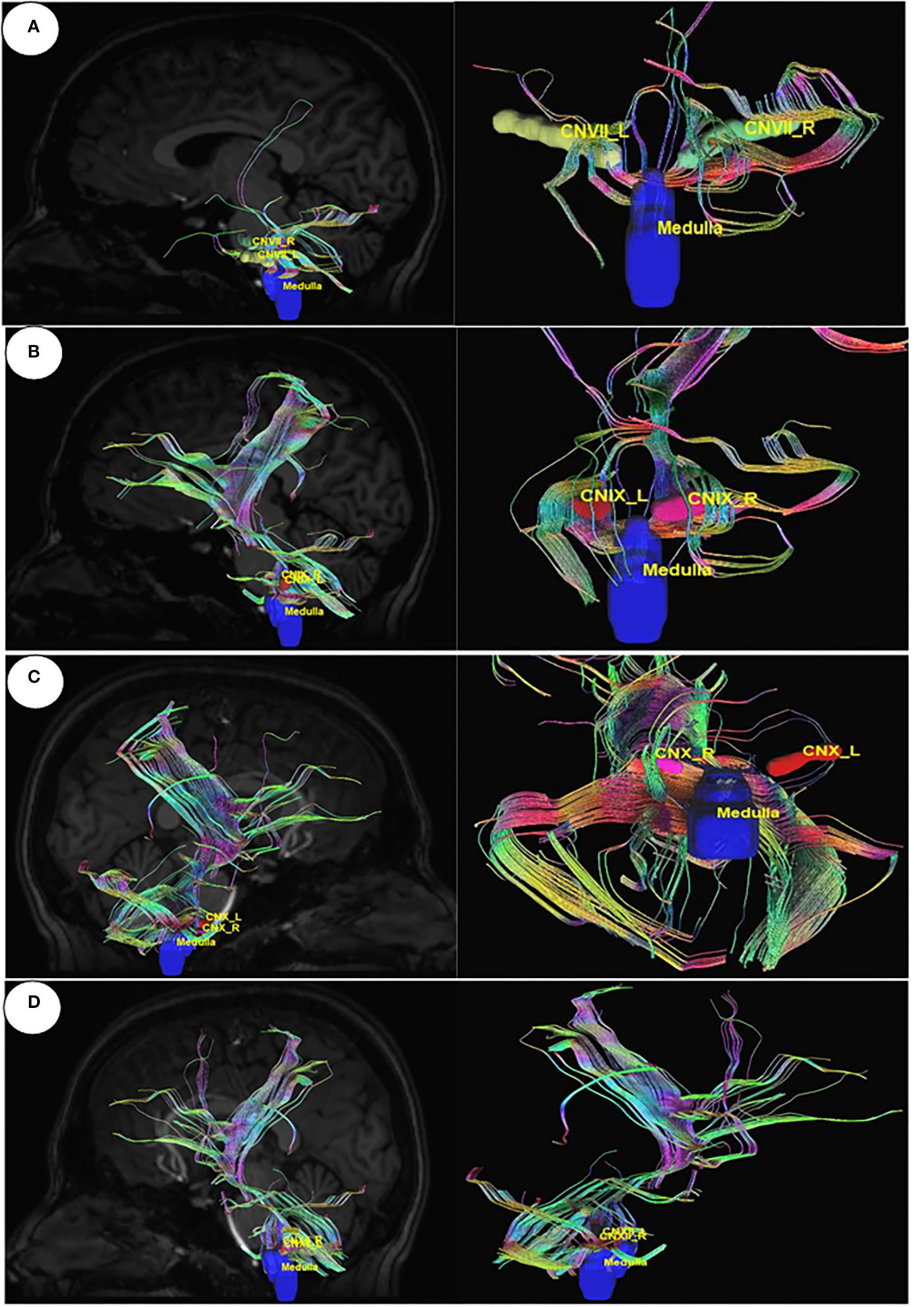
Diffusion MRI fiber tracking connecting medulla oblongata with gustatory nerves. (A) medulla to cranial nerve VII, (B) medulla to cranial nerve IX, (C) medulla to cranial nerve X, and (D) medulla to cranial nerve XI.
